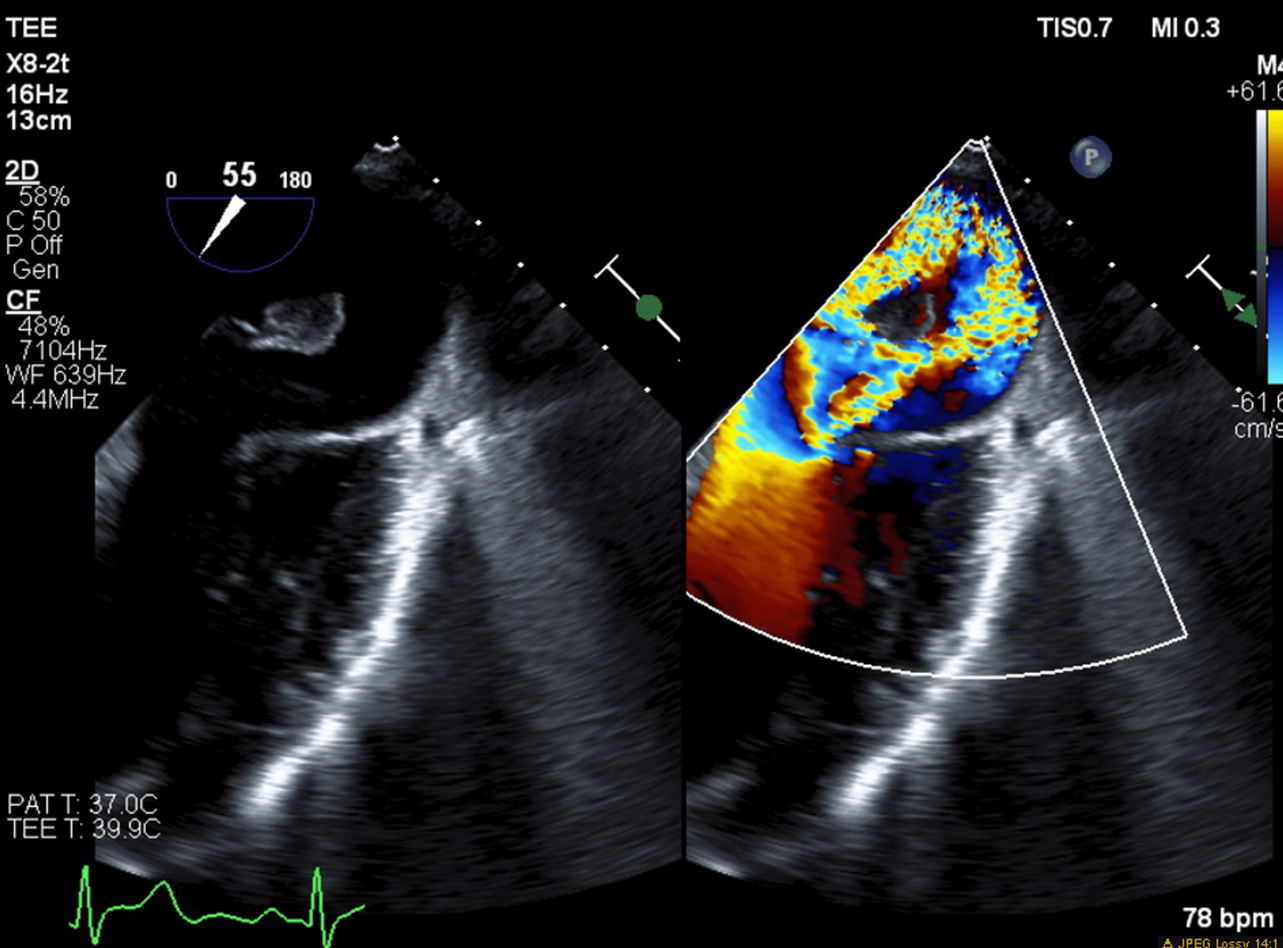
Transesophageal echocardiogram midesophageal two-chamber view showing flail papillary muscle and severe mitral regurgitation
